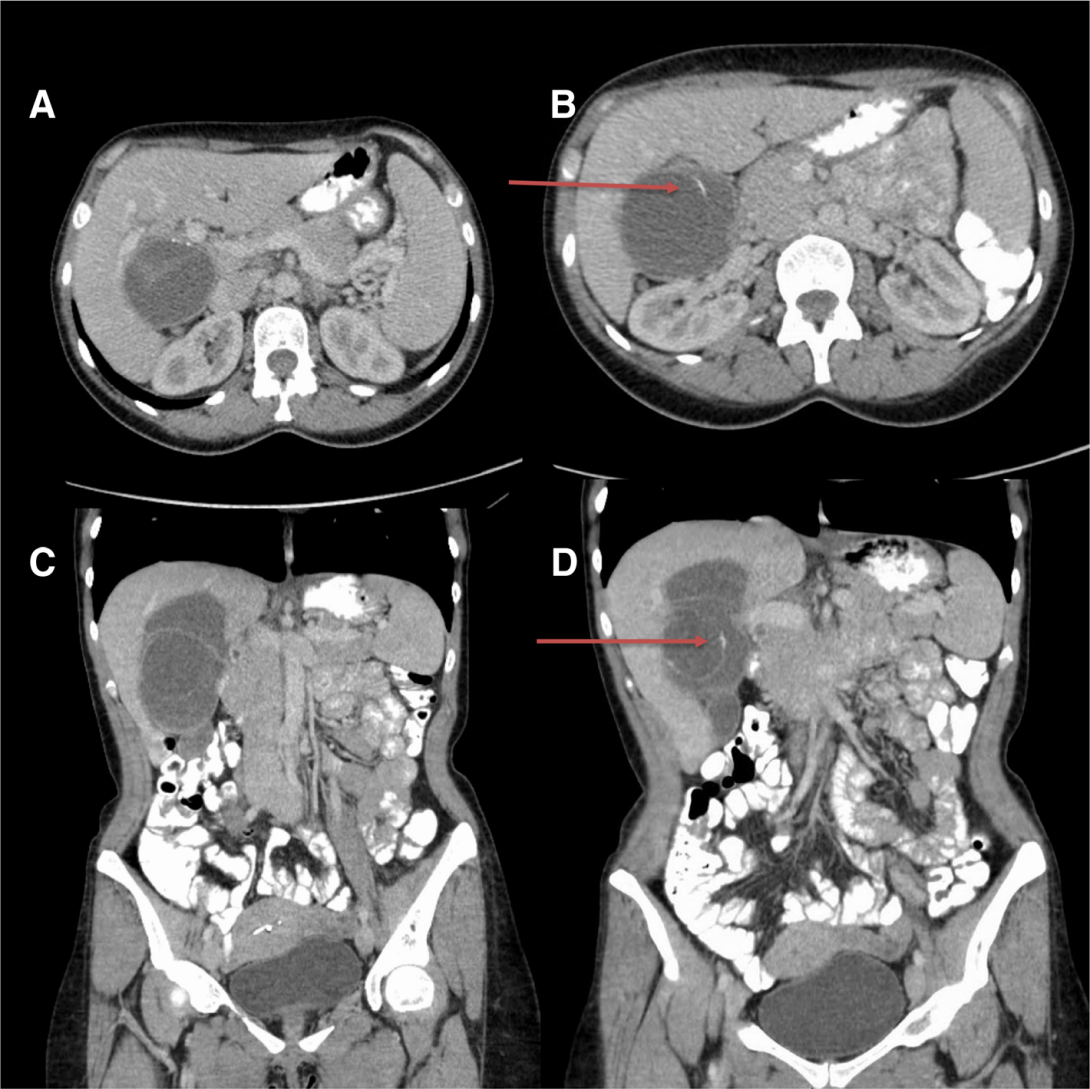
CT scan with oral and intravenous contrast in axial (1a and 1b) and coronal (1c and 1d) views, demonstrating a multi-loculated cystic mass embedded within segments V and VIII of the liver and causing deviation of the right portal vein. Arrows pointing towards the partially calcified septations
